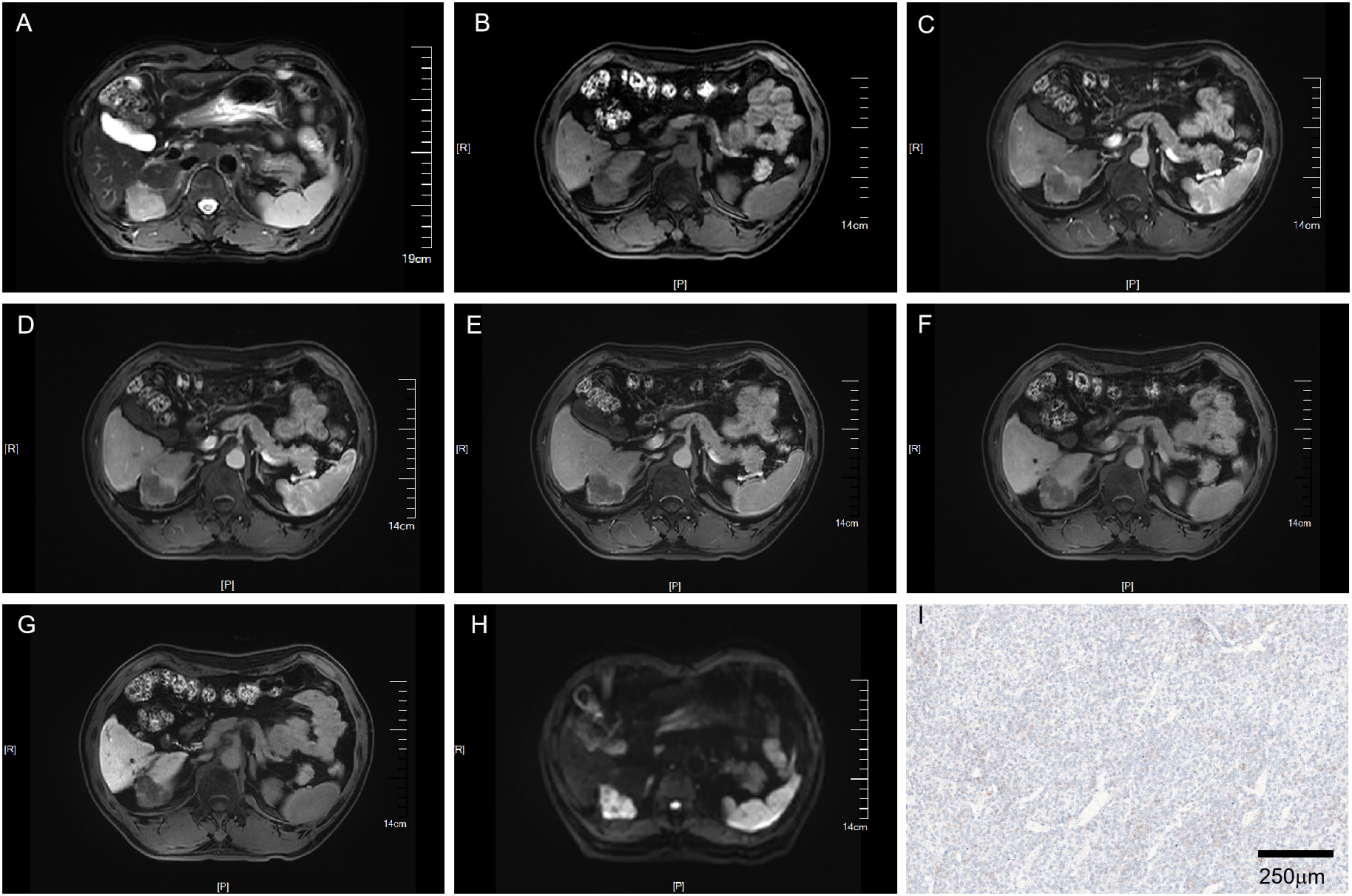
MRI of a VEGFR2-negative HCC patient with LI-RADS 3. Patient summary: a 59-year-old man with HCC in the right lobe of the liver, AFP level 2.7ng/ml, lesion dimension on MRI 3.8cm, and LI-RADS score of 3. (A) Axial T2-weighted MRI. (B) Axial T1-weighted MRI. (C, D) Early (15s) and late arterial phase (25s). (E) Portal venous phase (1min). (F) Delay phase (3min). (G) Hepatobiliary phase (15min). (H) Axial diffusion-weighted MRI. (I) VEGFR2-negative in pathology. MRI, magnetic resonance imaging; LI-RADS, liver imaging reporting and data system; AFP, alpha-fetoprotein; VEGFR2, vascular endothelial growth factor receptor 2.
